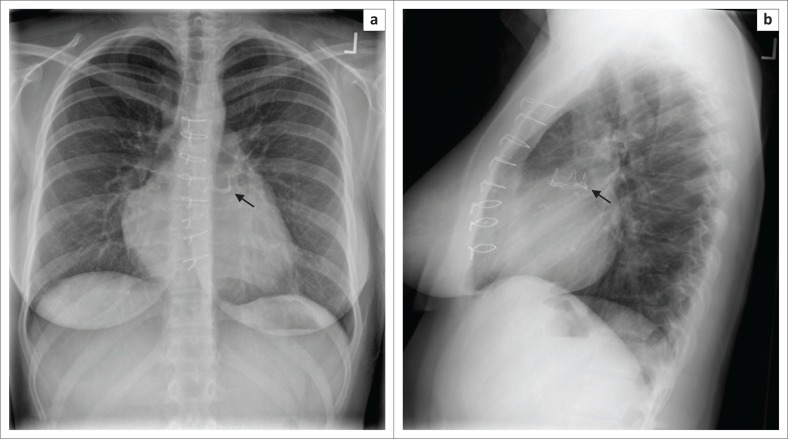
Frontal (a) and lateral (b) chest radiographs (CXRs) showing prosthetic pulmonary valve replacement (arrows).
